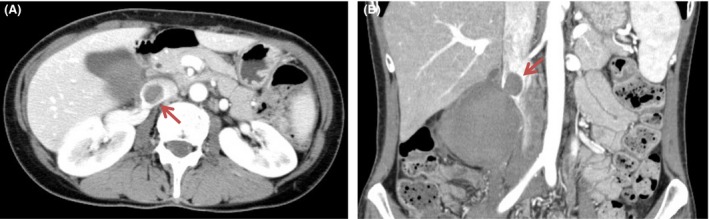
After 4 days, Enhanced CT revealed the proliferation of the thrombus, although there was no remarkable change in the mass and there was proliferation of the thrombus.
